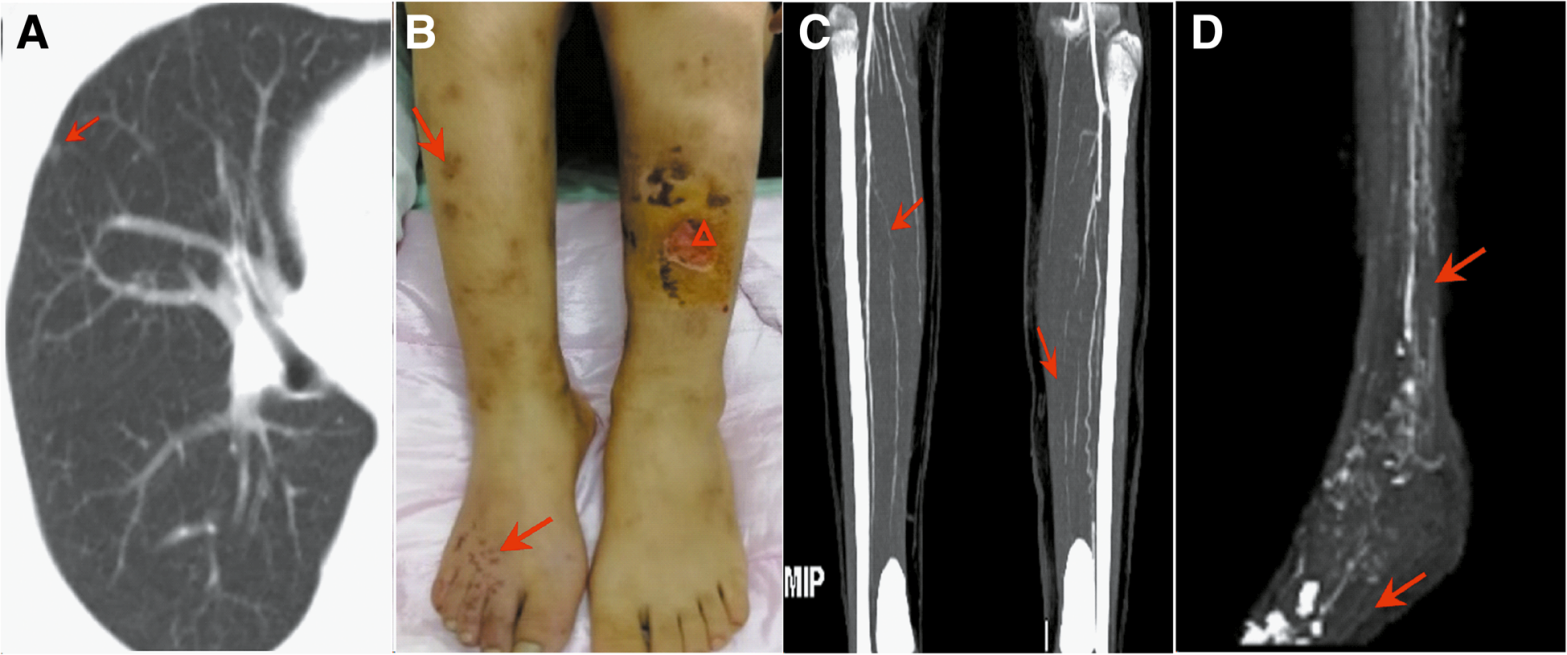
a: The chest CT scan showed that a change of ground-glass opacities and nodule was found on the middle lobe of the right lung (red arrow) b: Skin lesion presenting as purpura in lower limbs (red arrow), and an ulceration with purulent exudate, sized 3 cm * 4 cm in the left leg (triangle), c-d: The CTA of lower extremities showed extensive stenosis and/or occlusion of shank and foot arteries (red arrow)
